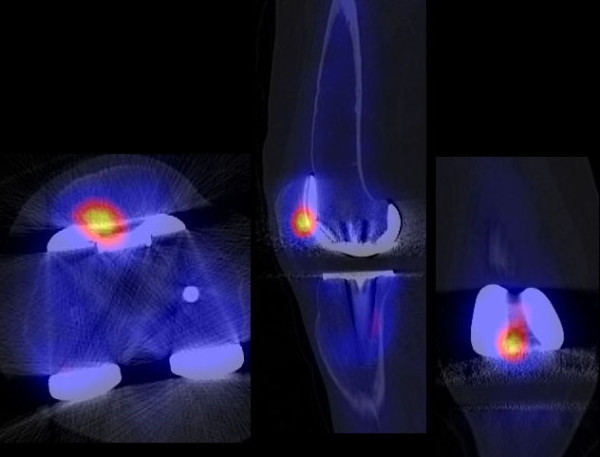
SPECT/CT imaging of a patient with patellofemoral problems as cause of painful total knee arthroplasty.
